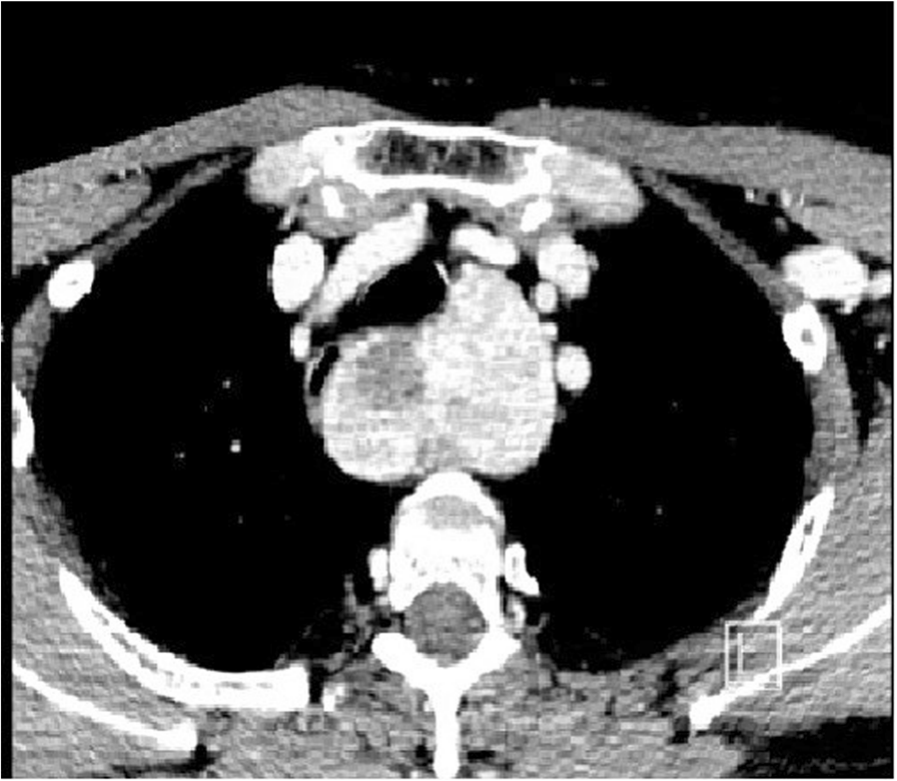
CT image of a substernal goiter that deepens beneath the aortic arch
